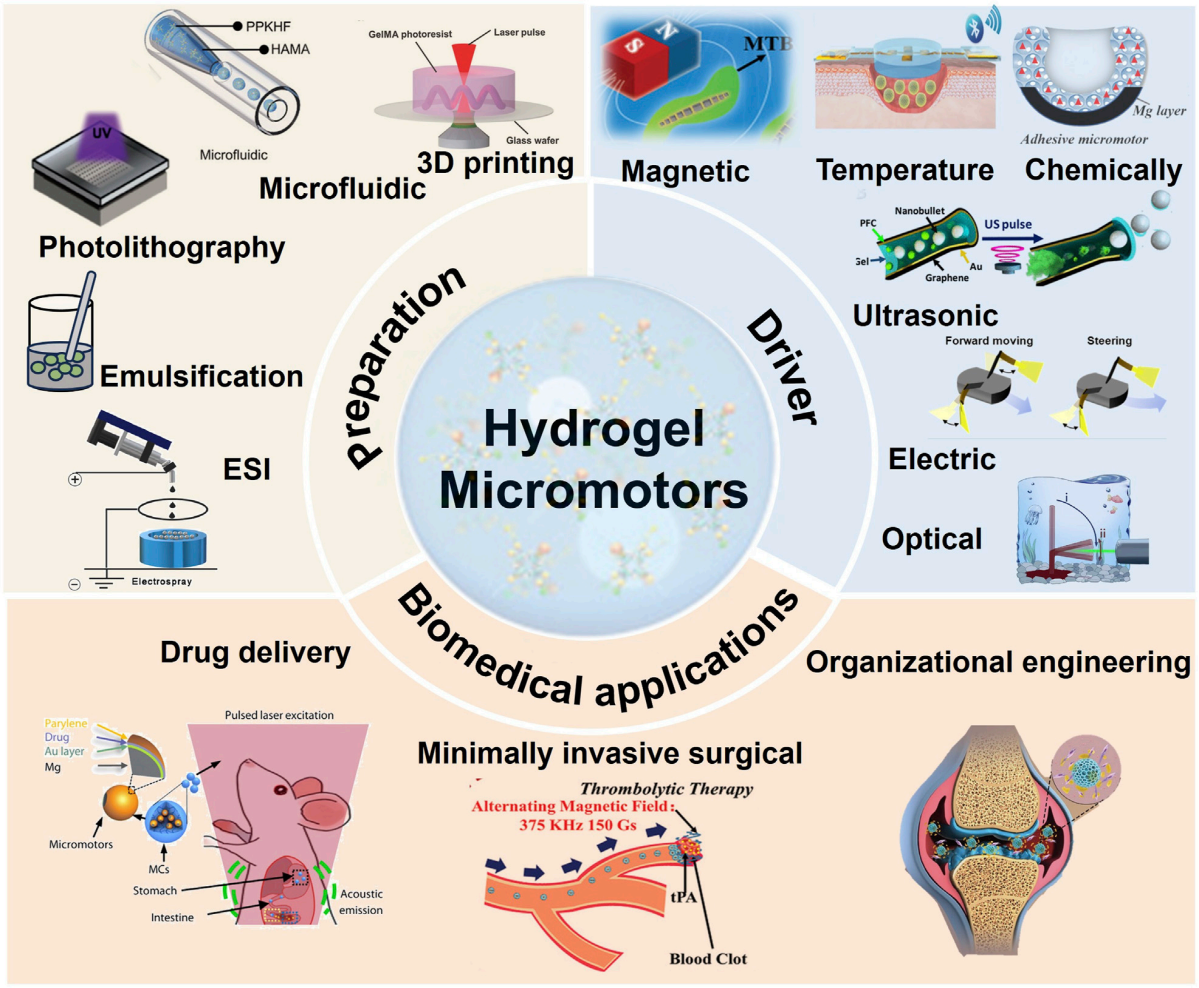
Preparation, driver and biomedical applications of hydrogel micromotors (Soto et al., 2016; Wang et al., 2018; Wu et al., 2019; Zhao et al., 2019; Zhong et al., 2019; Kim et al., 2020; Pang et al., 2020; Xie et al., 2020; Li et al., 2021; Cai et al., 2022; Ko et al., 2022; Yao et al., 2023; Bai et al., 2024). 3D printing, Copyright ^©^ 2018, John Wiley and Sons Microfluidic, Copyright ^©^ 2023 The Authors. Advanced Science published by Wiley-VCH GmbH Photolithography, Copyright ^©^ 2020, John Wiley and Sons ESI, Copyright ^©^ 2021 Acta Materialia Inc. Published by Elsevier Ltd. All rights reserved. Magnetic, Copyright ^©^ 2020, John Wiley and Sons Temperature, Copyright ^©^ 2020 The Authors. Published by WILEY-VCH Verlag GmbH and Co. KGaA, Weinheim Chemically, Copyright ^©^ 2021 The Authors. Advanced Science published by Wiley-VCH GmbH Ultrasonic, Copyright ^©^ 2016, American Chemical Society Electric, Copyright ^©^ 2022, The American Association for the Advancement of Science Optical, Copyright ^©^ 2019, The American Association for the Advancement of Science Minimally invasive surgical, Copyright ^©^ 2020, John Wiley and Sons Organizational engineering, Copyright ^©^ 2023, John Wiley and Sons.
